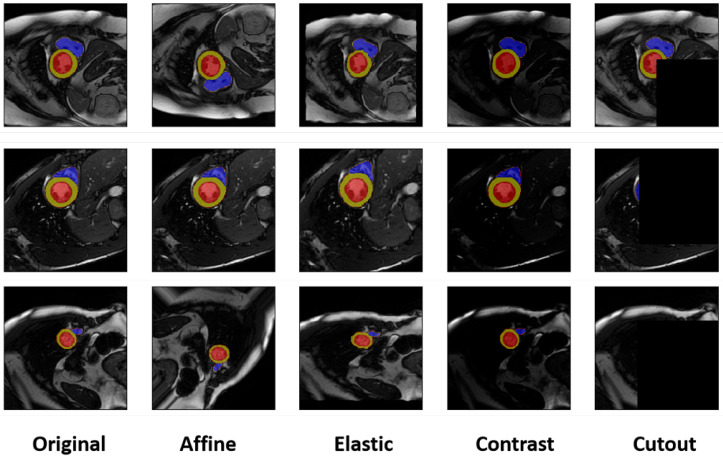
Visualizations of comparative data augmentation methods applied to cardiac MRI images.
